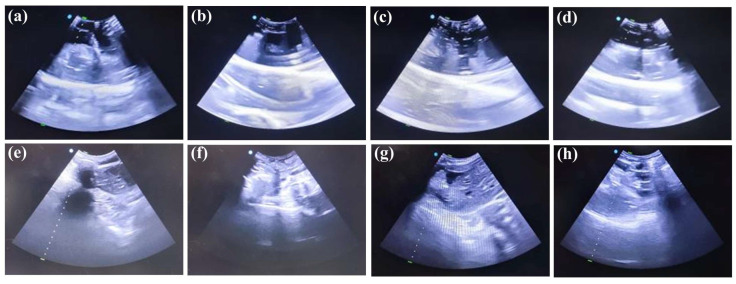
Contrast-enhanced ultrasound images of (a–d) silicone tubes in vitro and (e–h) rabbit liver in vivo after injection of saline and different nanobubbles: (a,e) saline; (b,f) PLLA nanobubbles; (c,g) PLLA@PDA nanobubbles; and (d,h) PLLA@PDA-DOX nanobubbles.
